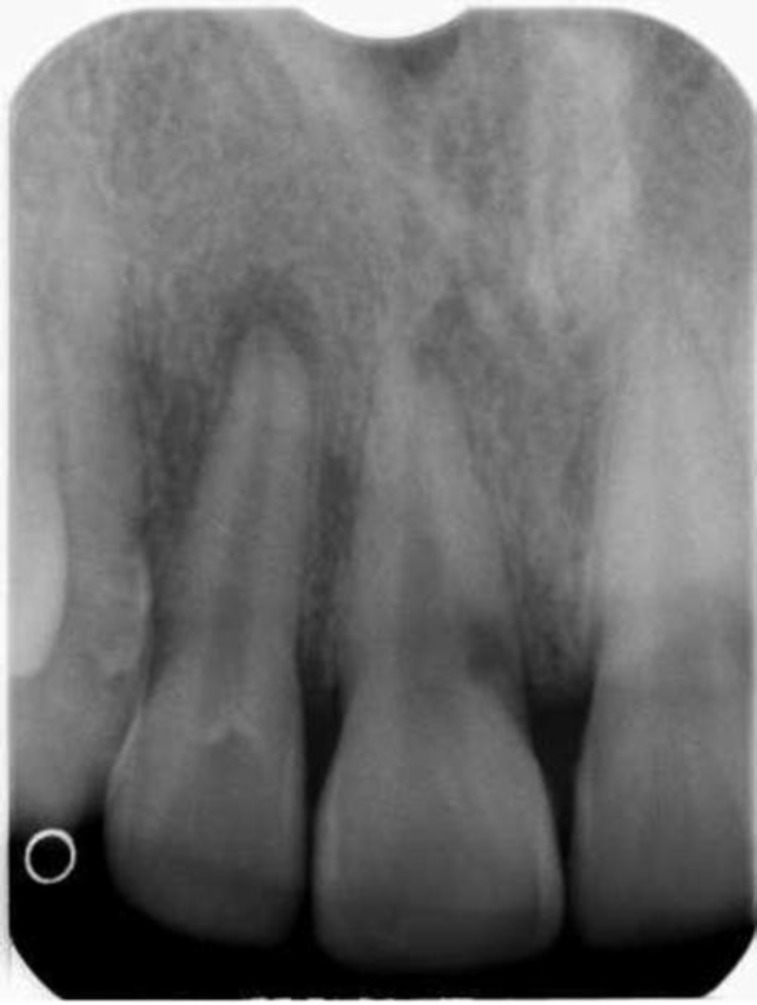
Pre-operative Radiograph
